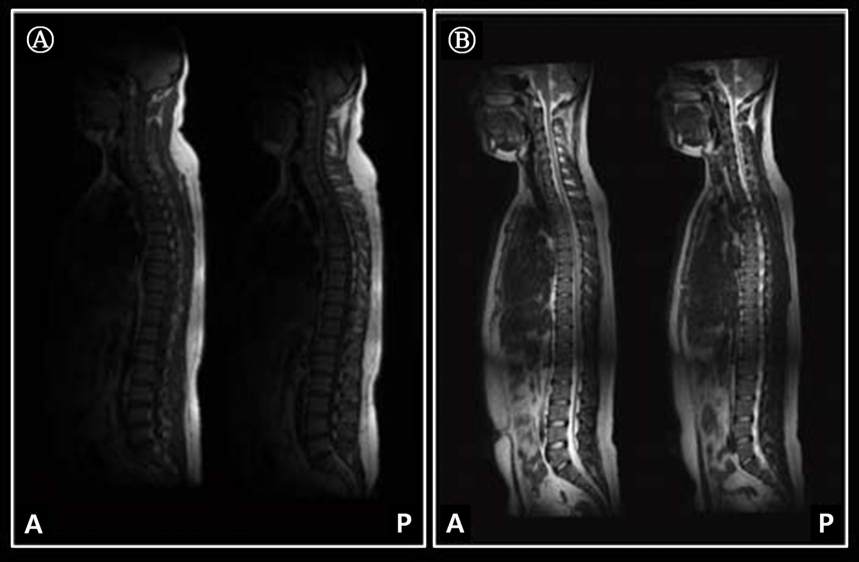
C-T-L spine MRI stitching result images (sagittal sections) using SIFT algorithm and developed GUI configuration for a representative subject. Datasets are acquired from 1.5 T MRI system (A) and 3.0 T MRI system (B).
